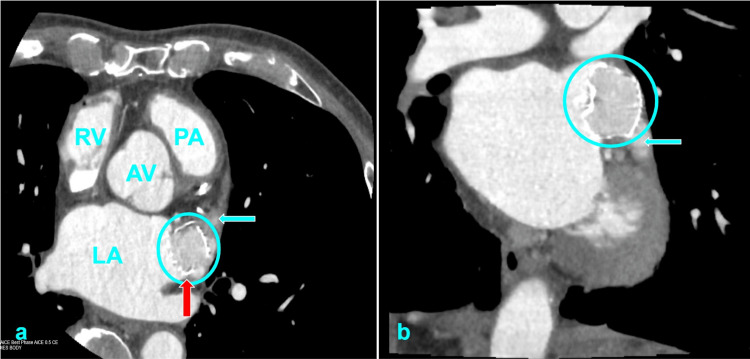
Cardiac computed tomography with contrast on surveillance imaging at day 45 after left atrial appendage occlusion (LAAO). Complete contrast opacification is noted within the LAAO device (blue circle) and left atrial appendage (LAA) (blue arrow in panels a & b), with a peri-device leak noted along the posterior edge of the LAA ostium (red arrow). Panel (a) shows the heart in the transverse plane and (b) in the coronal plane. LAAO: left atrial appendage occlusion, LAA: left atrial appendage, RV: right ventricle, LA: left atrium, AV: aortic valve, PA: pulmonary artery.
